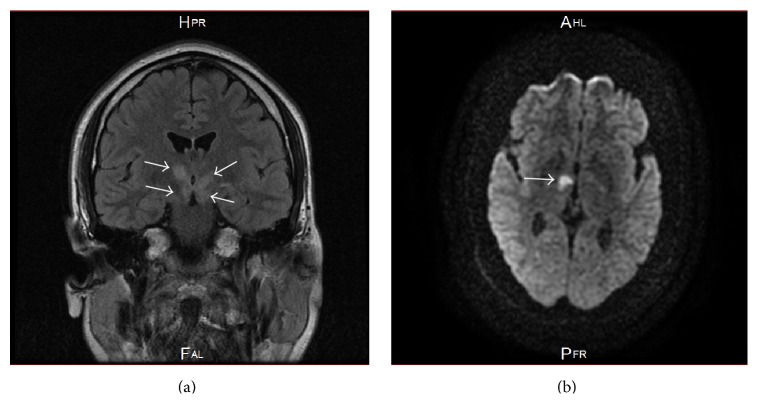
Images of the brain MRI study. (a) Coronal FLAIR-T2 showing bilateral pathological hyperintense signal abnormalities in periventricular, periaqueductal area, in both crura cerebri, and in both hypothalami (arrows). (b) Axial diffusion weighted imaging DWI showing a right thalamic infarct as a hyperintense signal.
